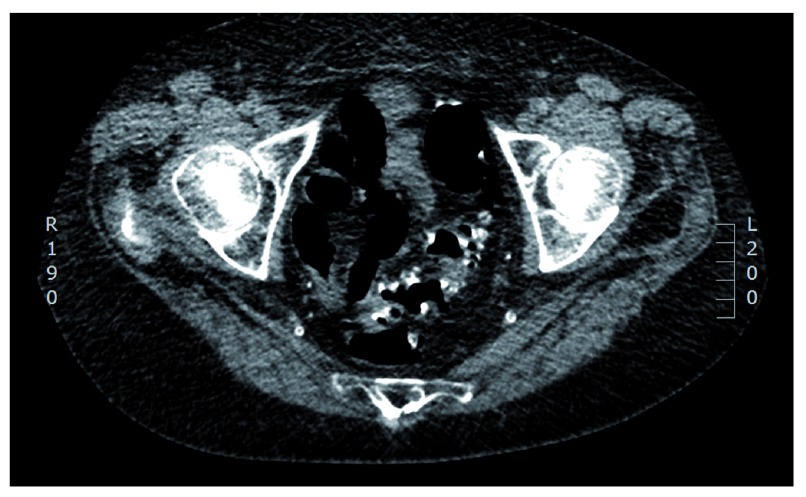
CT colonography depicting extensive diverticular disease and narrowing of the distal sigmoid.
